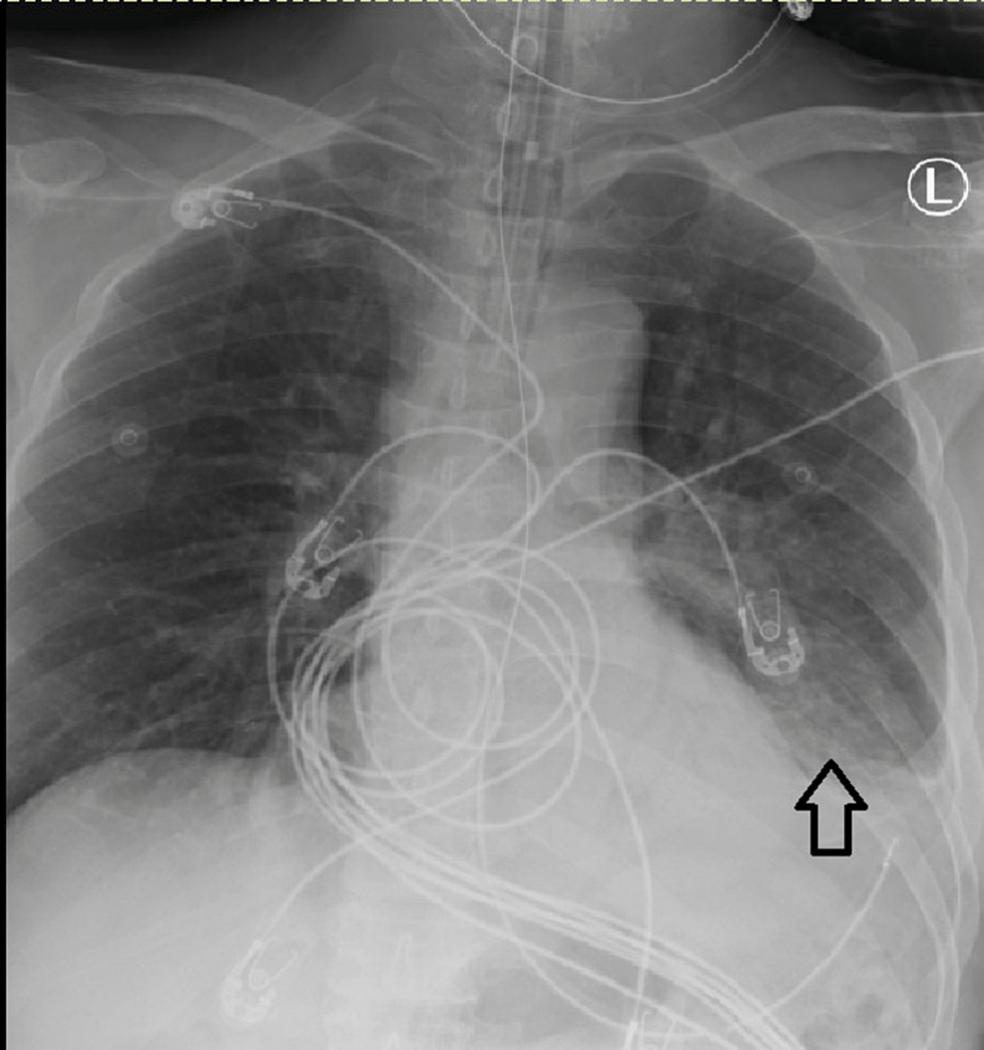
Chest X-ray A posterior-anterior chest X-ray showing a left-sided basal infiltrate (arrow).
